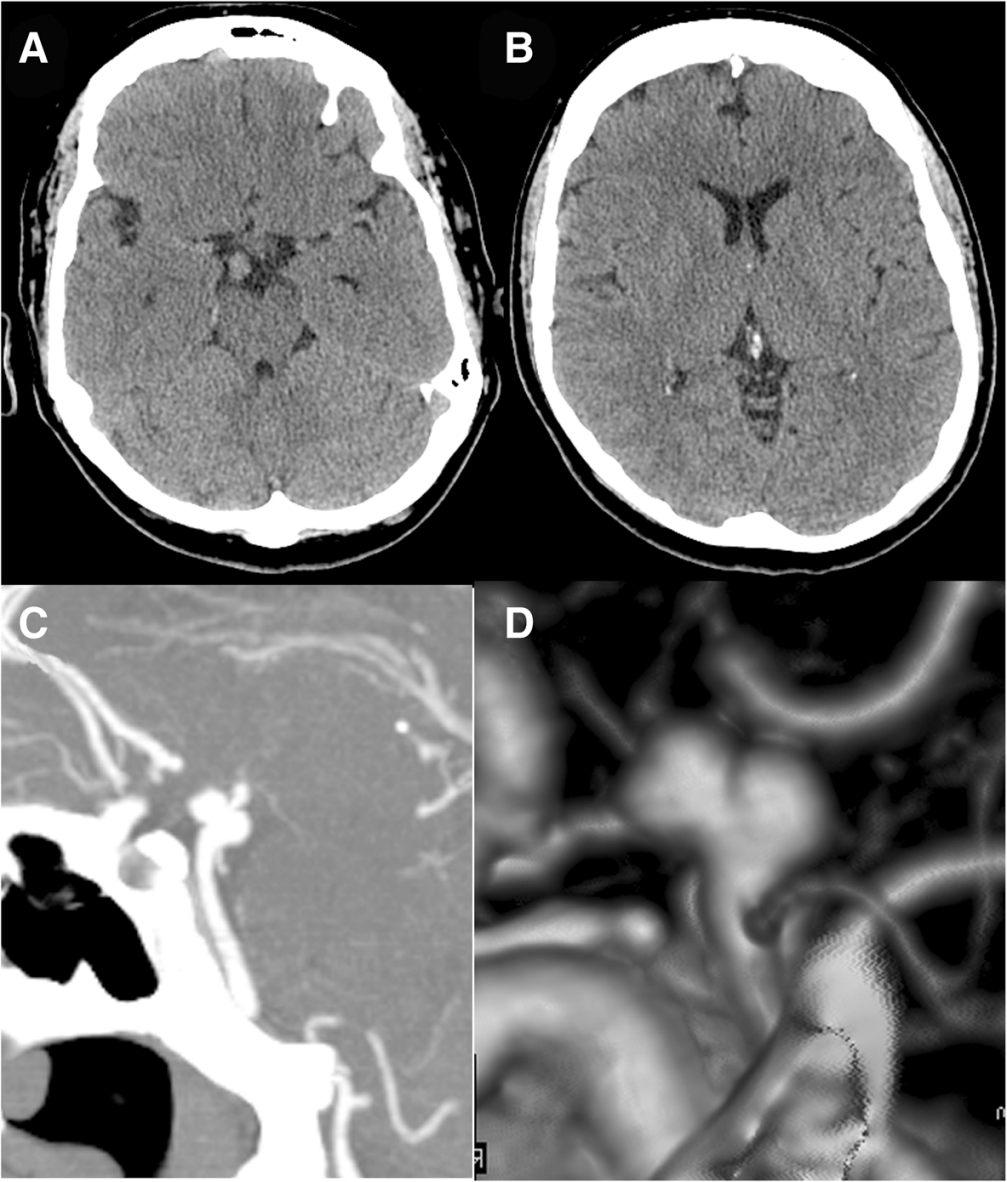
Computed tomography at first presentation. Cranial computed tomographic scan showing density in the right interpeduncular cistern (A) and no subarachnoid hemorrhage or hydrocephalus (B). A computed tomographic angiogram sagittal view (C) and lateral view of a reconstruction (D) show an aneurysm arising from the basilar artery distal to the origin of the right superior cerebellar artery.
